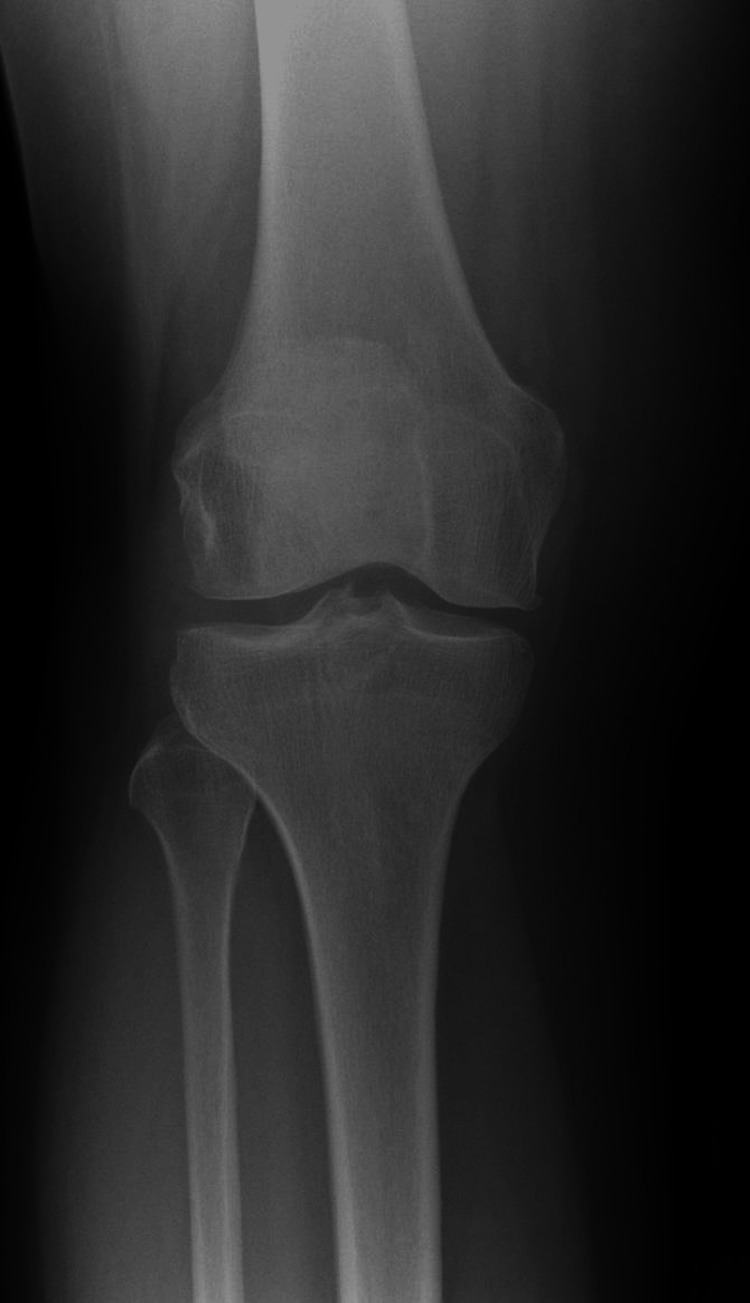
Representative radiograph of Kellgren-Lawrence grade 1 knee osteoarthritis Anteroposterior weight-bearing radiograph demonstrating minute osteophyte formation at the tibial margins and some joint-space narrowing. Findings correspond to Kellgren-Lawrence grade 1, representing early osteoarthritic change.
